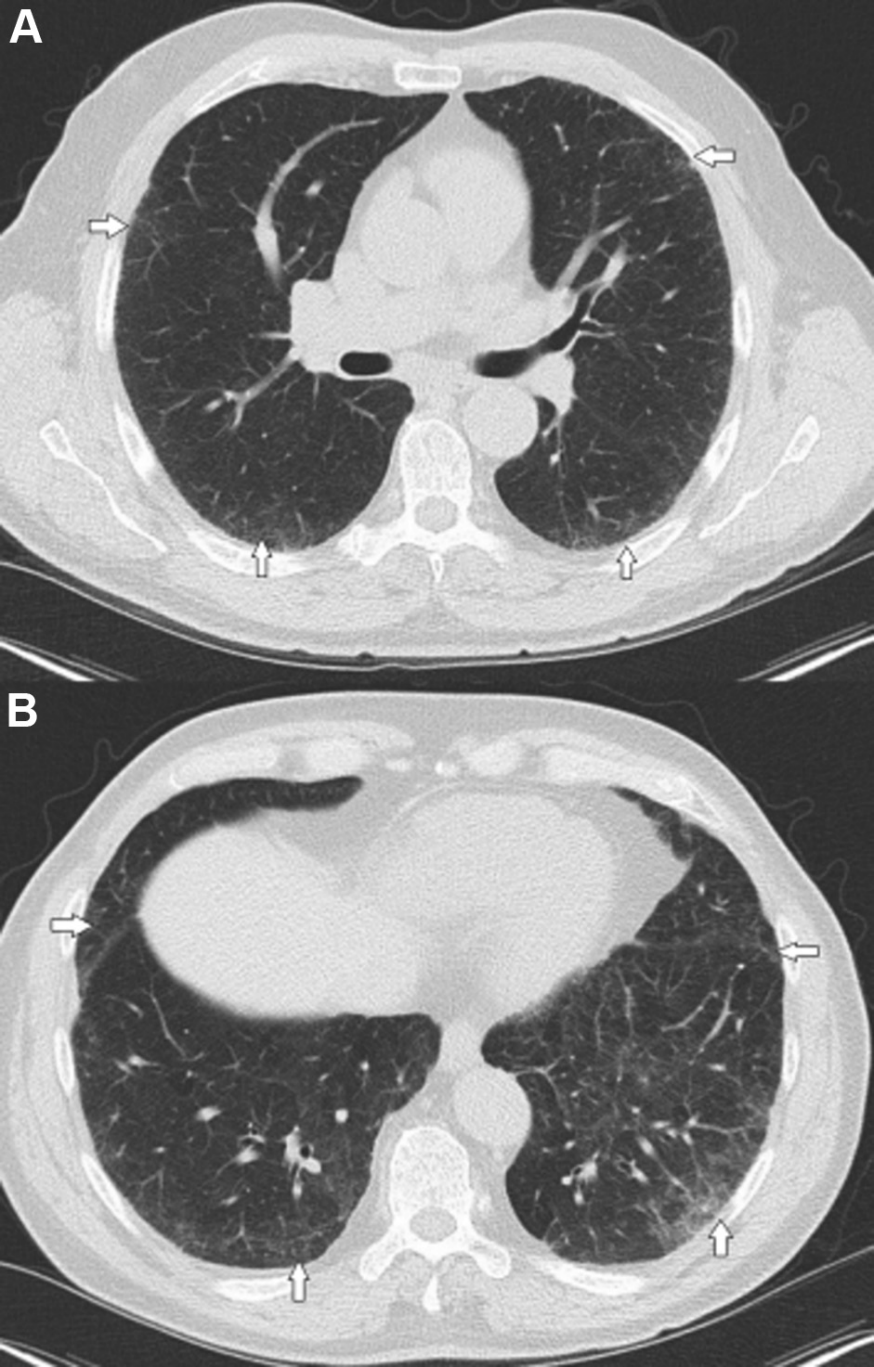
An example of axial images from the carina (A) and lung base (B) from a patient identified with interstitial lung abnormalities in the context of a chest CT scan ordered for routine cancer surveillance. White arrows highlight regions of subpleural reticulation. This chest CT scan is consistent with an indeterminate usual interstitial pneumonia pattern. The patient eventually developed progressive pulmonary fibrosis based on lung function over a 2-year period and was ultimately started on antifibrotic therapy.
